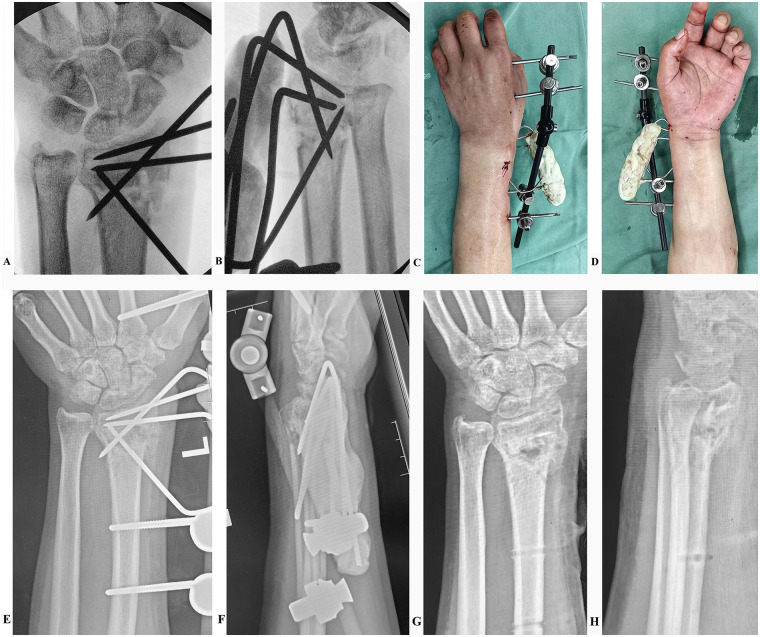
Immediately after surgery. (A) A PA X-ray. (B) A lateral X-ray. (C) A dorsal appearance of fixation. (D) An anterior appearance of fixation. (E) Bone healing shown on a PA X-ray after 6 weeks. (F) A lateral X-ray. (G) A PA X-ray taken 2 months after surgery. (H) A lateral X-ray.
